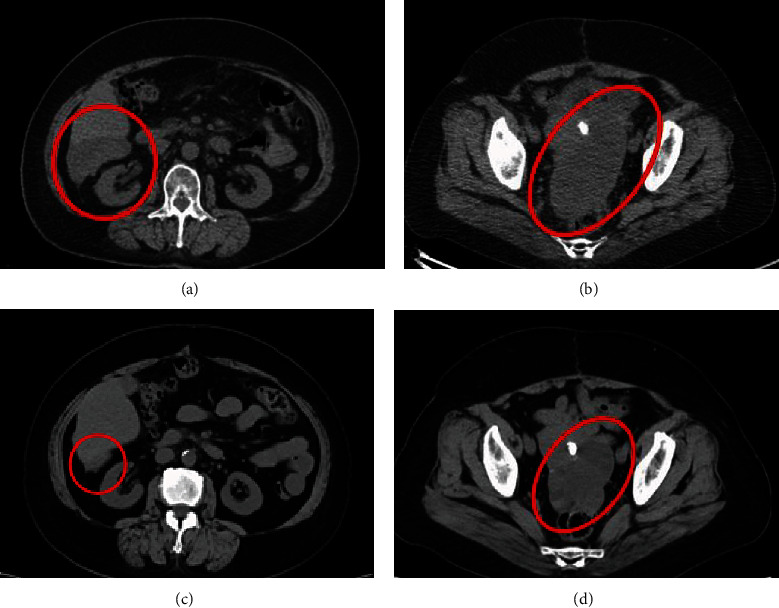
(a, b) Computed tomography revealed peritoneal dissemination of the surface of the liver and Douglas' pouch. (c, d) After four months of olaparib administration, computed tomography revealed a decrease in the size of the tumors.
